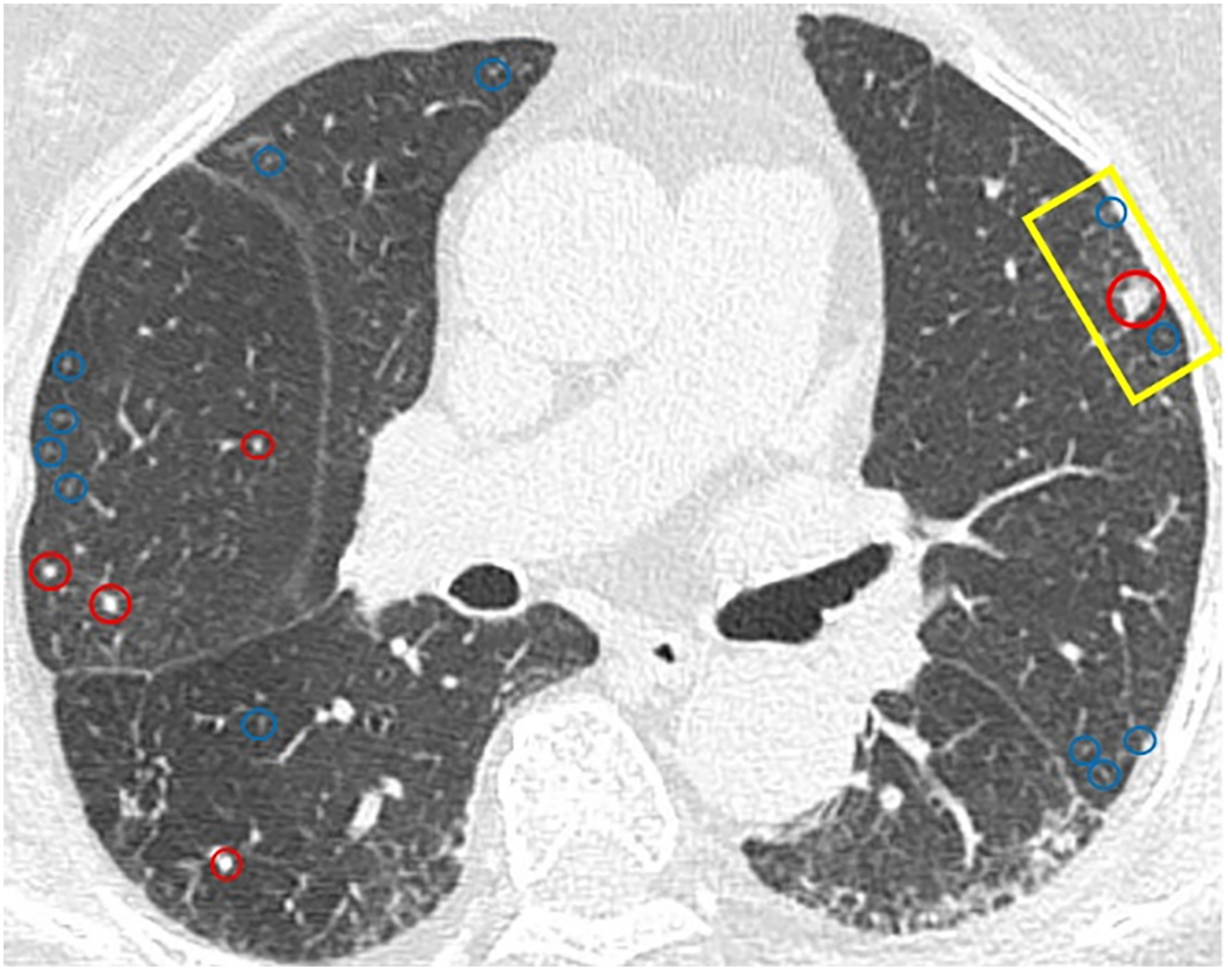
Axial slice of the chest computed tomography; areas marked with red circles represent solid nodules, and those marked with blue circles denote ground‐glass nodules. The territory marked by the yellow square is targeted for biopsy.
